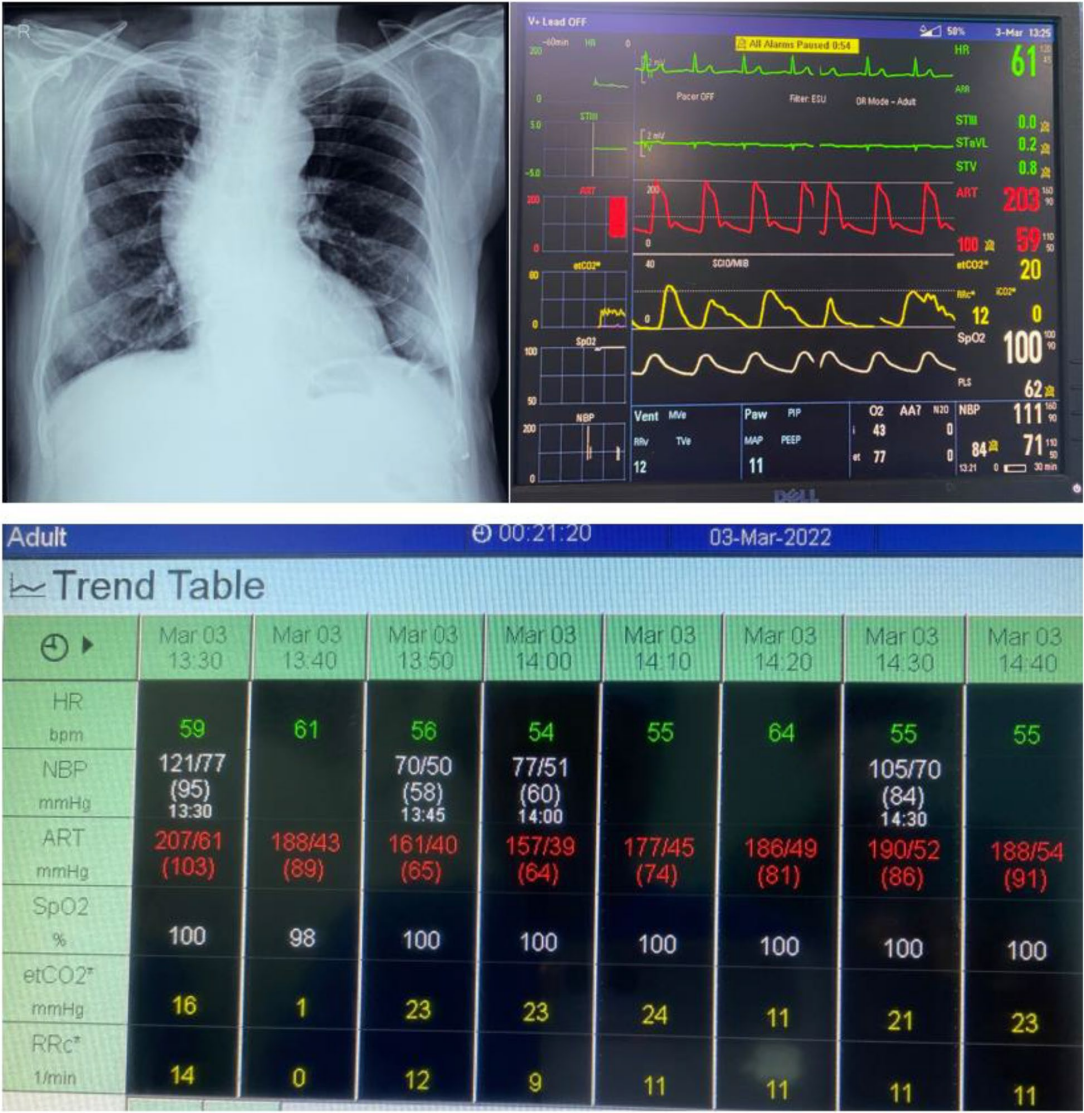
Chest radiograph with dilatation of the aorta (upper left); Monitor screen showing the disparity in blood pressures between both the upper limbs (upper right); Trend table depicting the intraoperative hemodynamic changes over time (bottom row)
